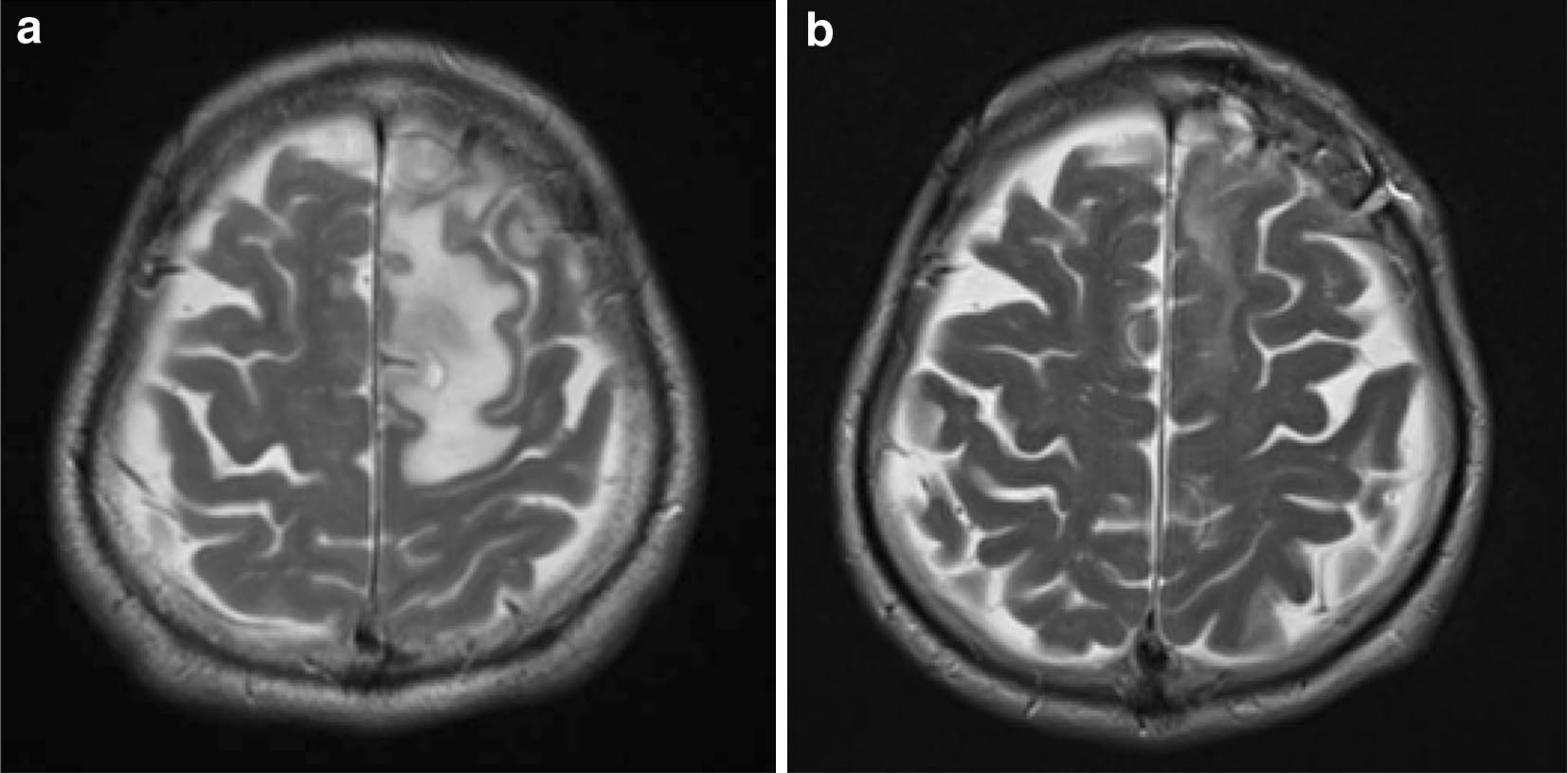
MRI scans of the brain on admission and 3 weeks after the initiation of therapy. An MRI scan on admission a showed high signal intensity on T2-weighted images in the white matter of the left temporal and right frontal lobes. Obvious mass effects were not observed. Contrast enhancement was seen along the brain surface and cerebral sulci; however, little enhancement of the brain parenchyma was detected. A follow-up brain MRI scan obtained 3 weeks after the initiation of antifungal and steroid therapy b showed overall improvement with a marked decrease in the size of the white matter lesions
